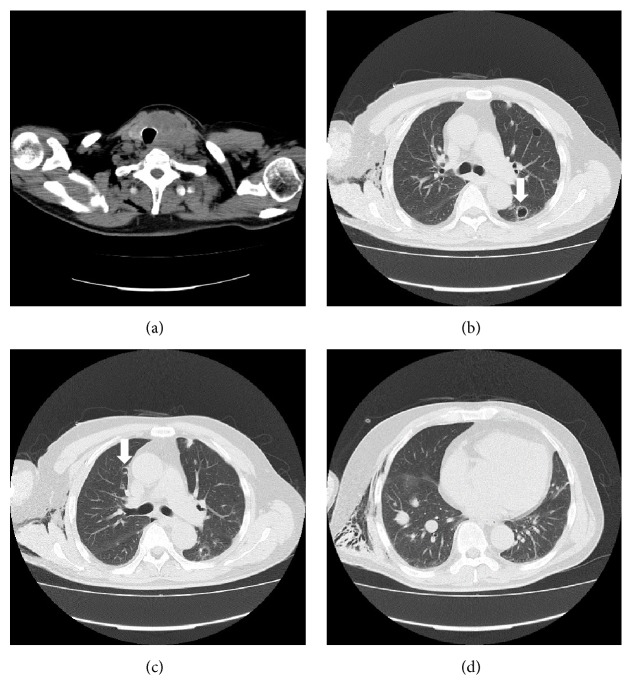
(a–d) Computed tomography on day 73 of lenvatinib start showed that primary tumor was stable disease and there is no recurrence of pneumothorax. Thin wall cavitations were observed in the metastatic lesions (arrow).
